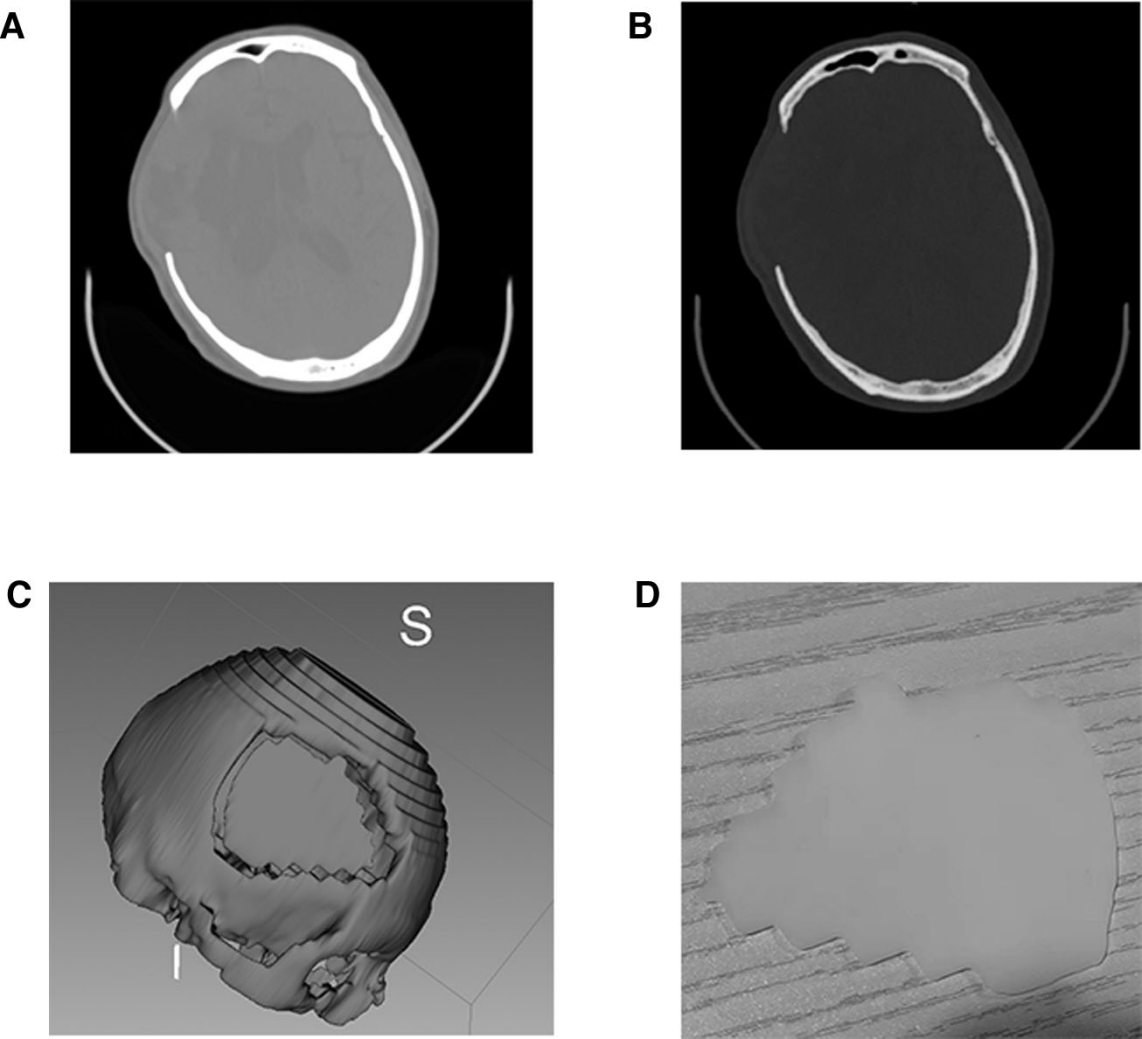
3D printed skull and mold from high resolution CT scan data. (A,B) High resolution CT scan data; (C) virtual mold in 3D Slicer; (D) 3D printed skull.
